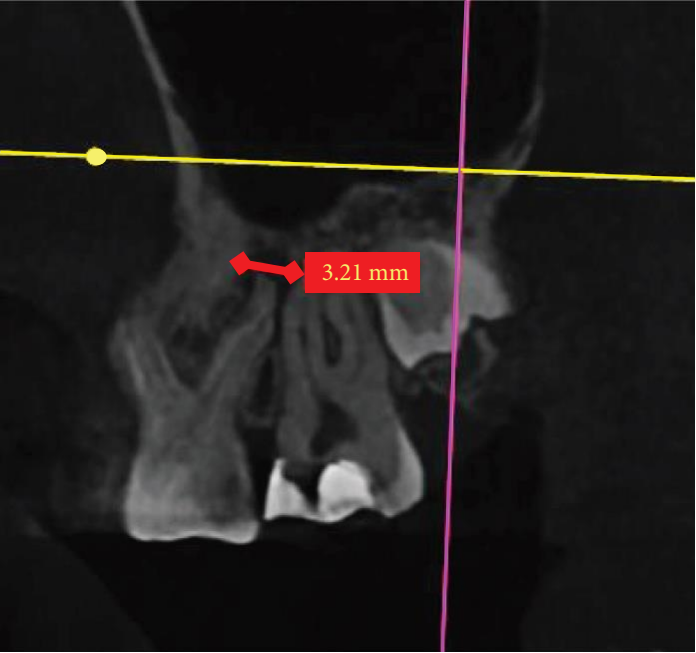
Periapical lesion extension in the sagittal view.
